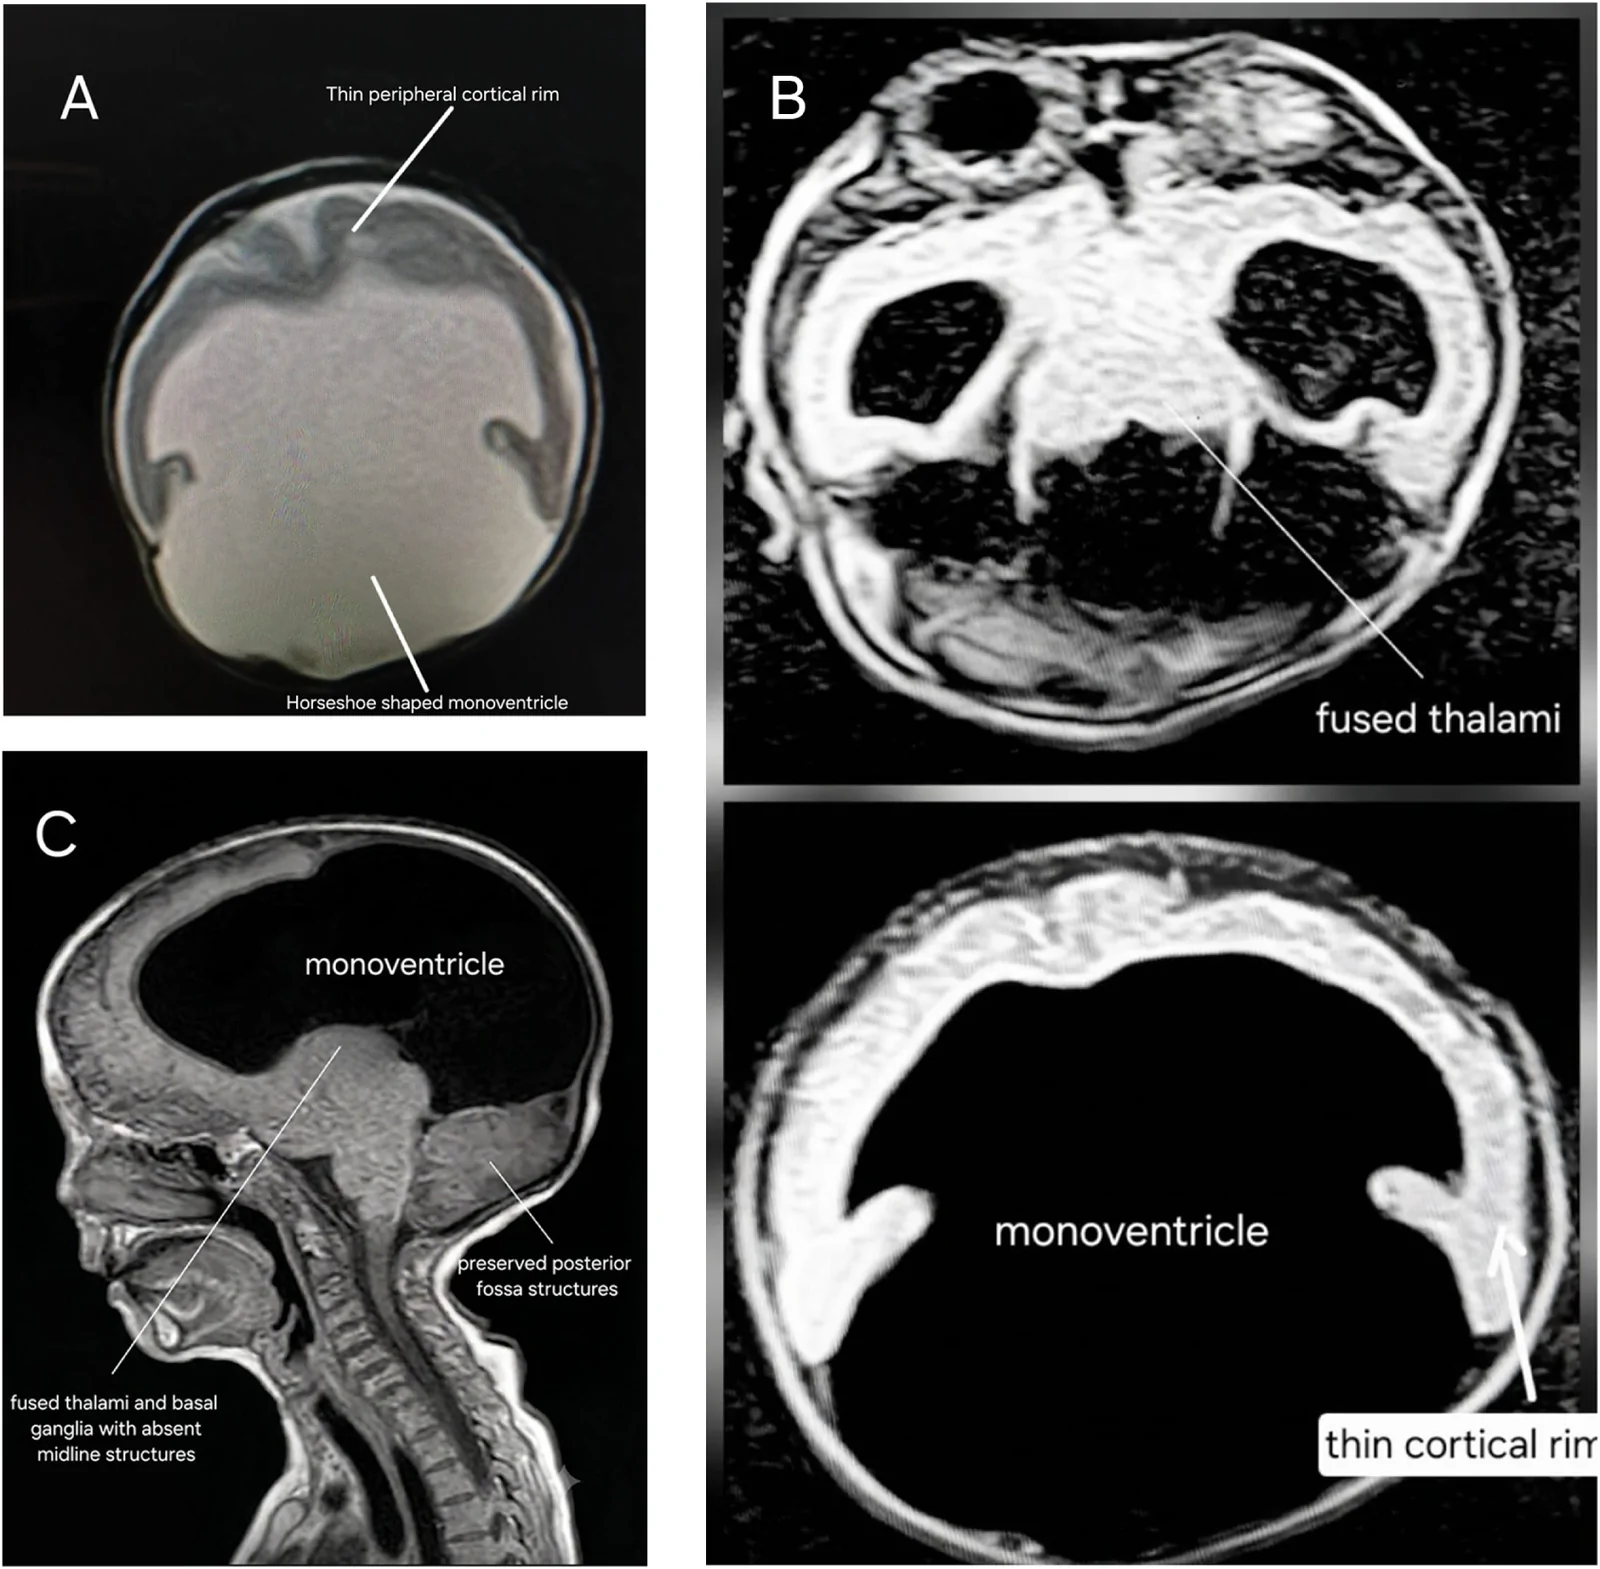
Brain MRI axial T2W (A) and axial FLAIR (B) sequences showing a large, horseshoe-shaped monoventricle with a thin peripheral cortical rim and complete suppression of CSF signal on FLAIR. The sagittal (C) image demonstrates fused thalami and basal ganglia at the midline with absence of the septum pellucidum and anterior falx cerebri, characteristic of the semilobar variant.
